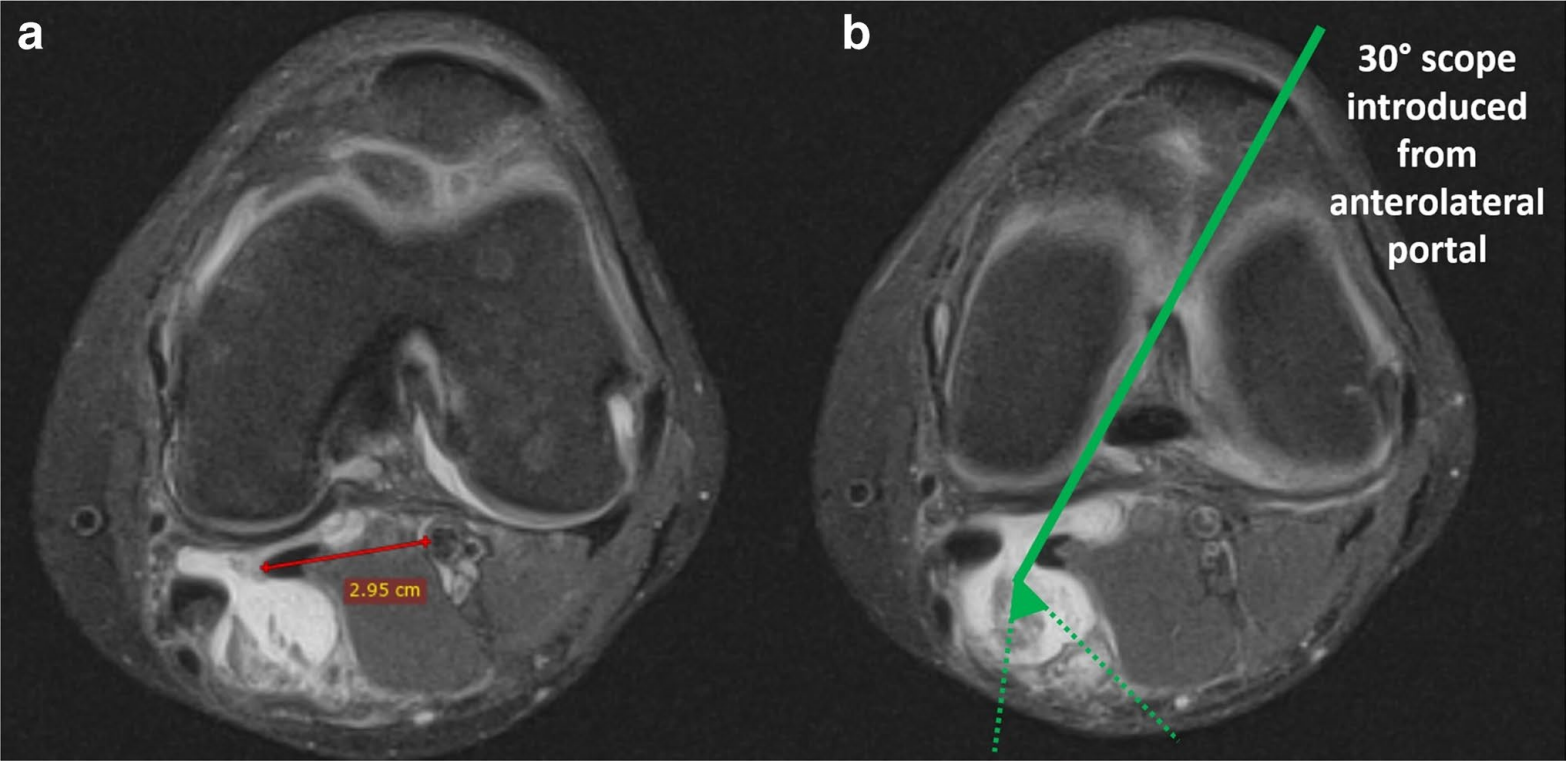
Transverse MRI scans of the left knee concerning spatial relationship between the popliteal cyst and neurovascular bundle: (a) in case of typical popliteal cyst localization in the gastrocnemius–semimembranosus bursa, the neurovascular bundle is protected by the medial head of gastrocnemius. (b) As can be seen, if the surgeon is working only within tissues possible to visualize from anterolateral portal after trans-notch maneuver, the neurovascular bundle is out of the surgical field
